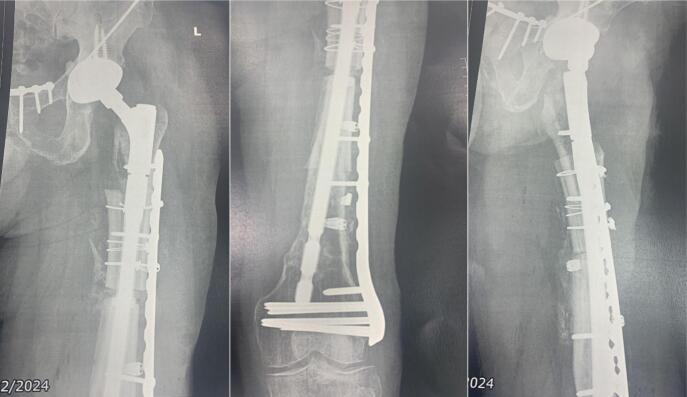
AP and lateral radiograph of the left femur three months post-op showing integration and stabilization of the alloprosthesis with signs of graft resorption at the proximal femur.
